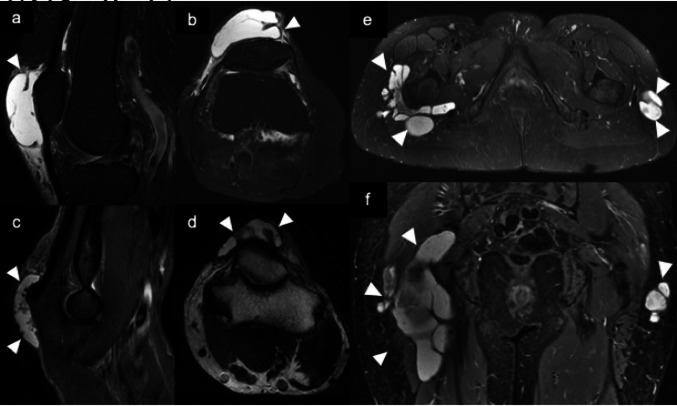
a, b Prepatellar bursitis. Axial (a) and sagittal (b) fat-suppressed T2-weighted MR images show a high signal intensity mass (arrow heads) located anterior to the patella. c, d Olecranon bursitis. Axial fat-suppressed T2-weighted (c) and sagittal T2-weighted MR images (d) demonstrate a flat-shaped cystic mass (arrowheads) located between the skin and underlying olecranon. d, e Trochanteric bursitis. Axial (e) and coronal (f) fat-suppressed T2- weighted images reveal a high signal intensity masses (arrowheads) surrounding the greater trochanter
